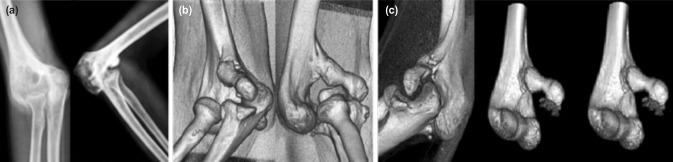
(a, b) AP and lateral radiograph and three dimensional reconstruction (3D) CT scan showing anterior dislocation of the elbow joint. (c) 3D CT reconstruction with subtraction of radius and ulna anteroposterior and lateral views demonstrating anatomy of the distal humerus.
